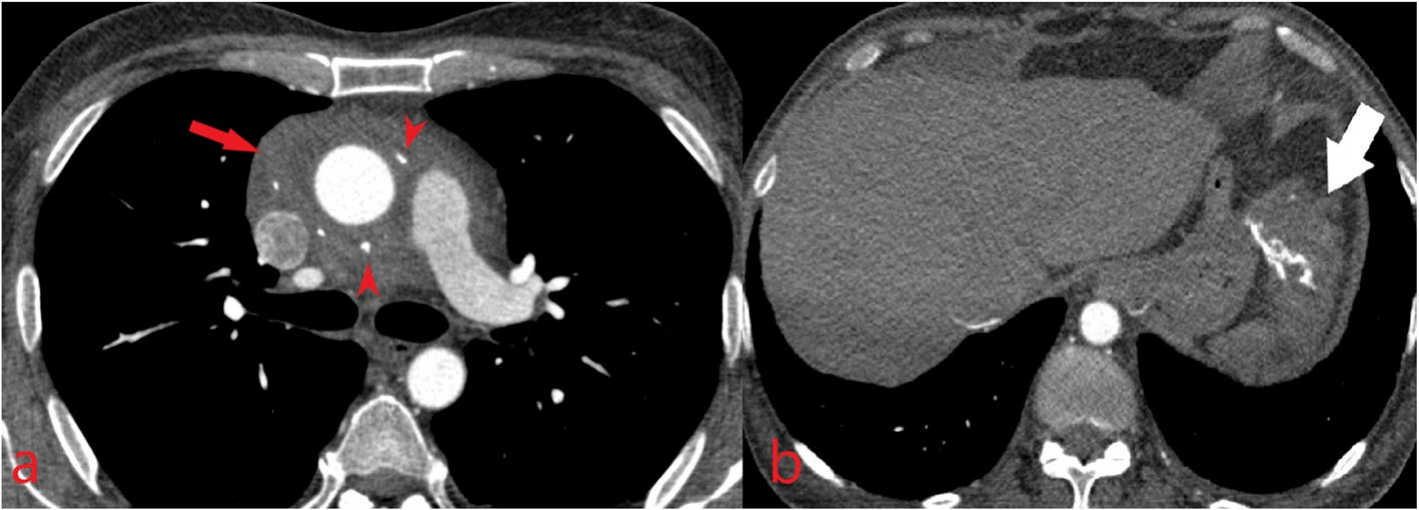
Contrast enhanced chest CT scan shows 3a: Mediastinal soft tissue infiltration (red arrow) with foci of calcification (red arrowhead) and 3b: Calcified soft tissue mass in upper abdomen (white arrow)
